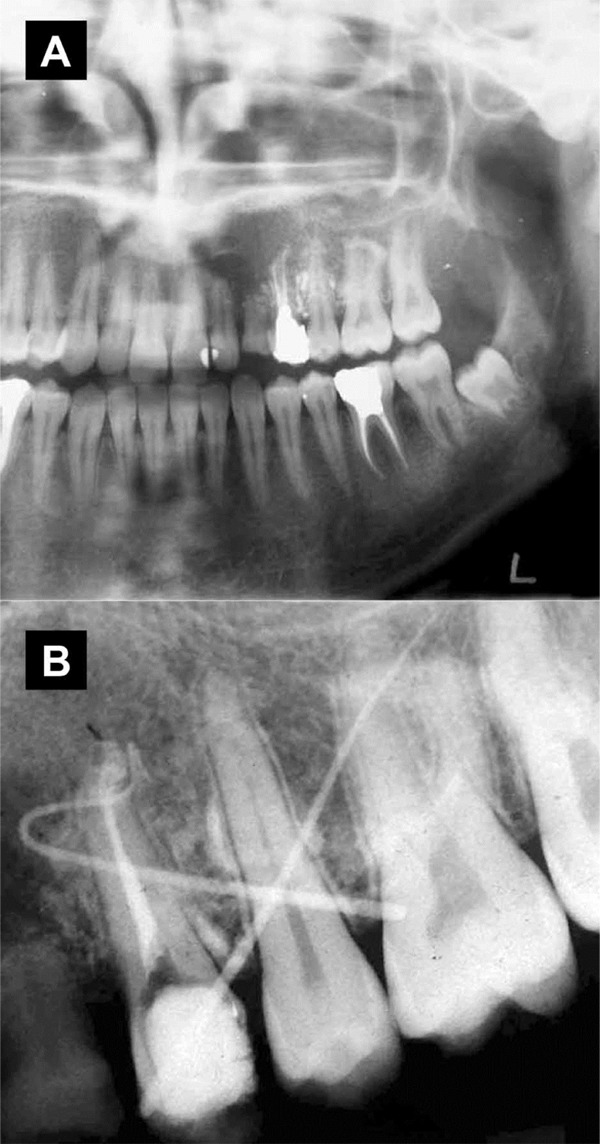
Radiographs showing radiopaque CaOH_2_ paste surrounding root of the premolars (A) and the tracing of gingival detachment by gutta-percha (B)
